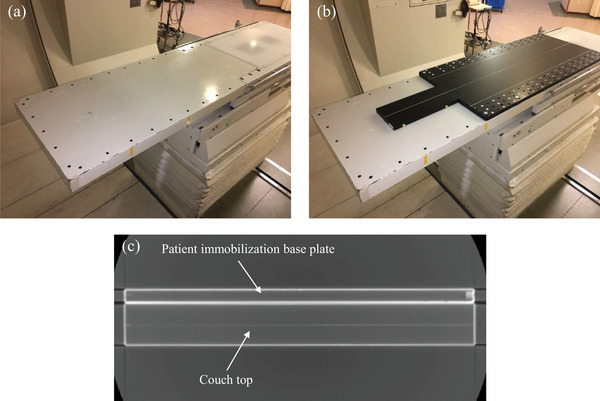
Overview of the (a) couch top and (b) patient immobilization base plate (PIBP) on the couch top (couch top plus PIBP). (c) Axial image of computed tomography of the couch top plus PIBP.
